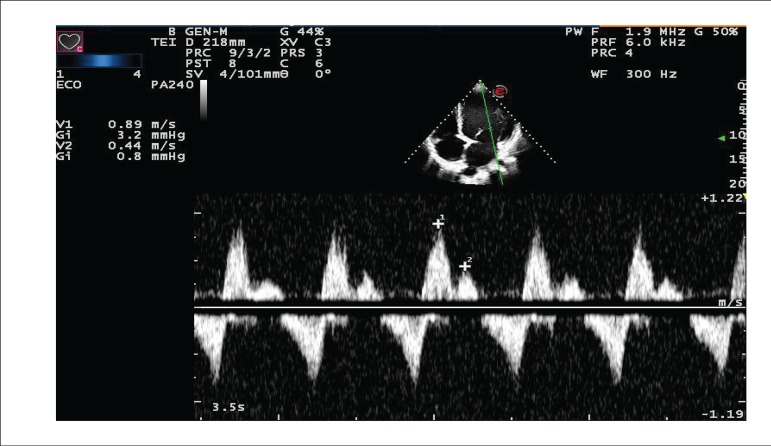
Echocardiogram. Restrictive ventricular filling.
